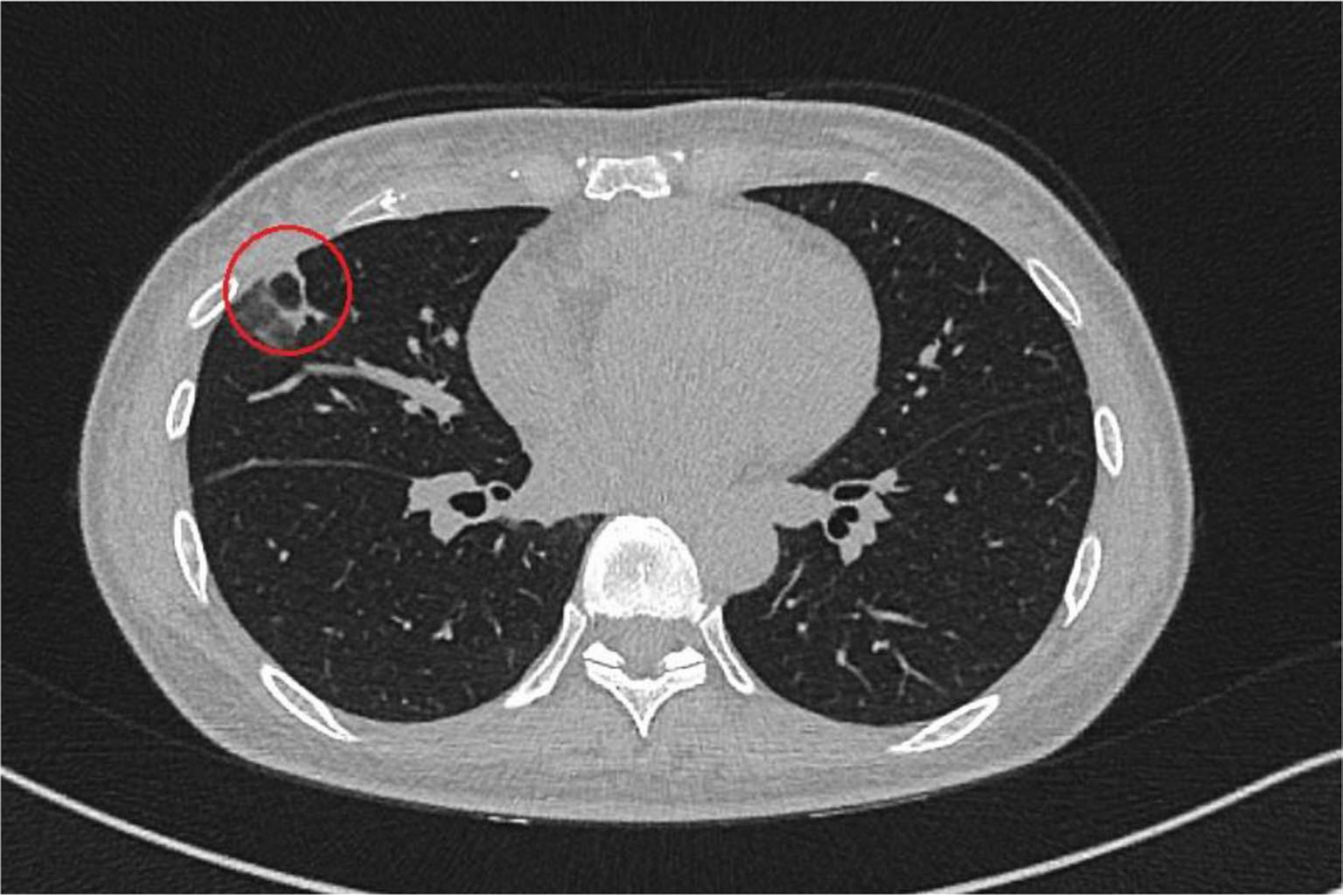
CT scan of the chest after three months of antifungal therapy (after 3 months of antifungal therapy, administered on an outpatient basis)
